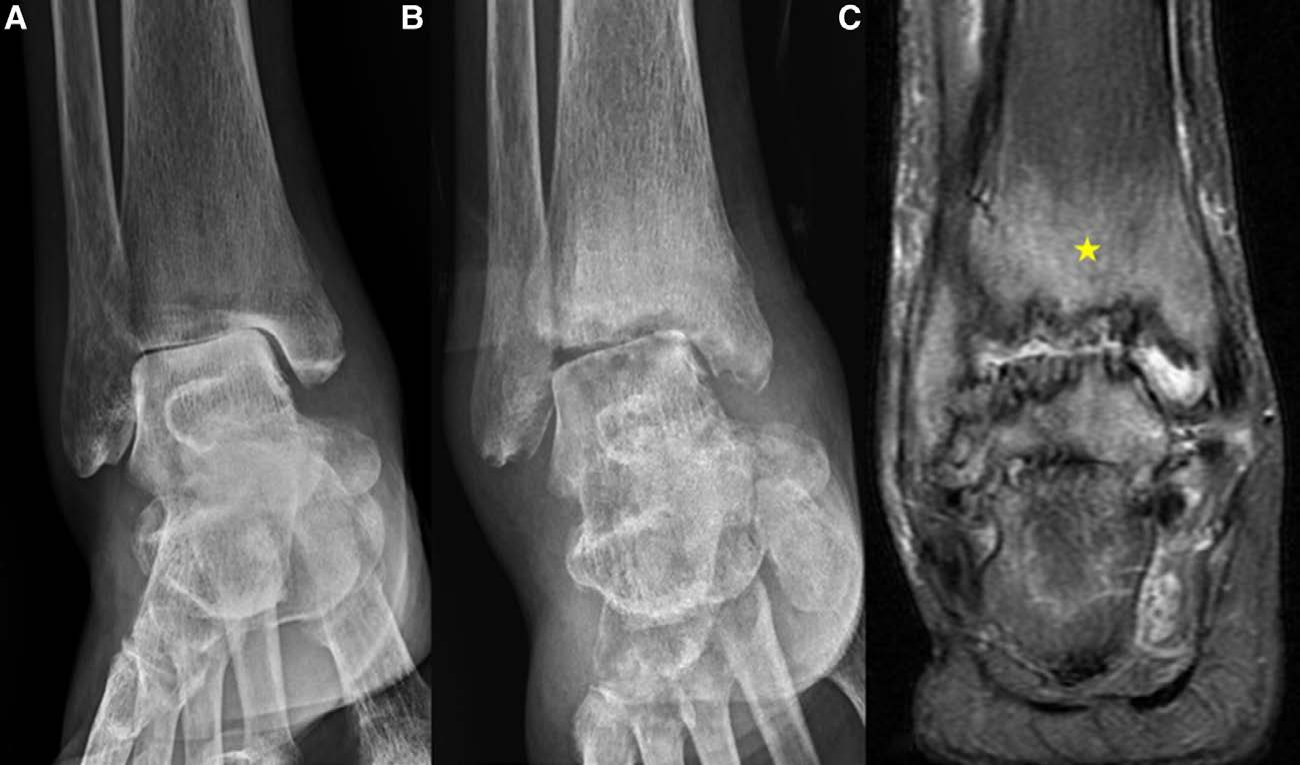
A series of image examinations including ankle radiography and magnetic resonance imaging in presented case: (A) The right ankle exhibited asymmetric joint space narrowing and minimal marginal osteophytosis during the initial admission. (B) Six months later, upon the second admission, new findings included subchondral bone erosion, pseudo-widened joint space, and surrounding soft tissue swelling. (C) T2-weighted imaging with fat suppression revealing bone erosion, surrounding bone marrow edema (star), small joint effusion, and conspicuous irregular synovial thickening.
